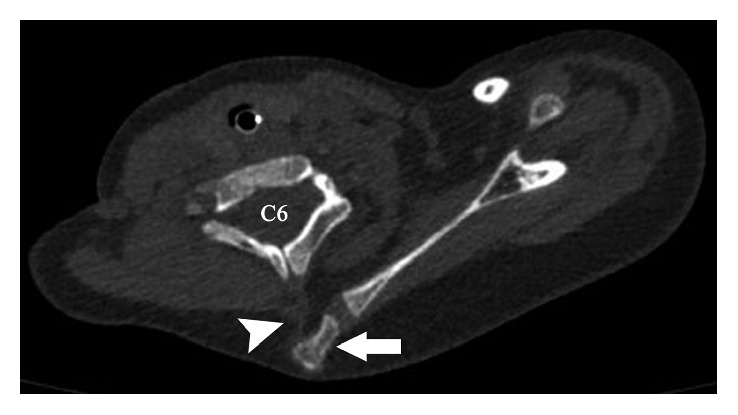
CT, axial image demonstrates Sprengel deformity with associated omovertebral bone (arrow) and fibrocartilaginous band (*a*rrowhead). (*Courtesy of Miguel Flores*, *MD*, *Orlando*, *FL.*)
